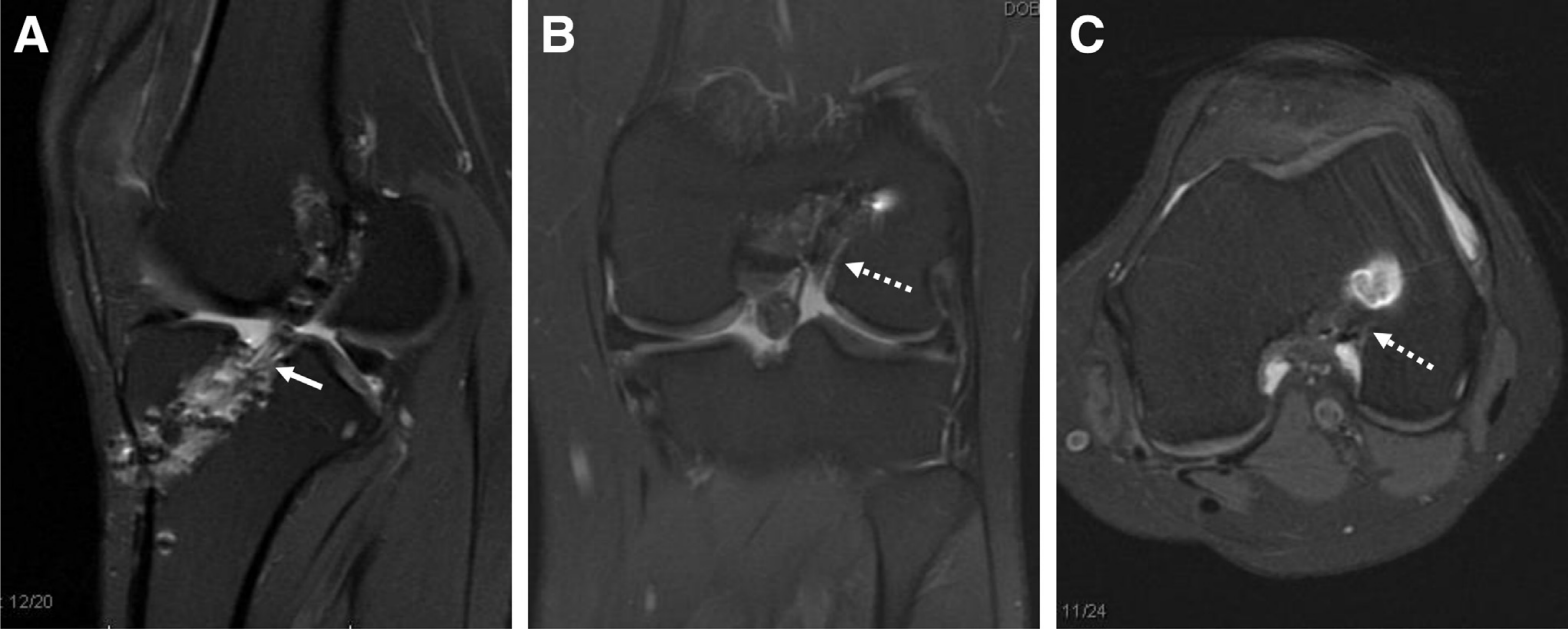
Preoperative magnetic resonance imaging (MRI) evaluation. Sagittal (A), coronal (B), and axial (C) T2-weighted MRI cuts of left knee showing a widened, posteriorly positioned tibial tunnel (solid arrow), as well as a widened and vertically malpositioned femoral socket (dashed arrows).
